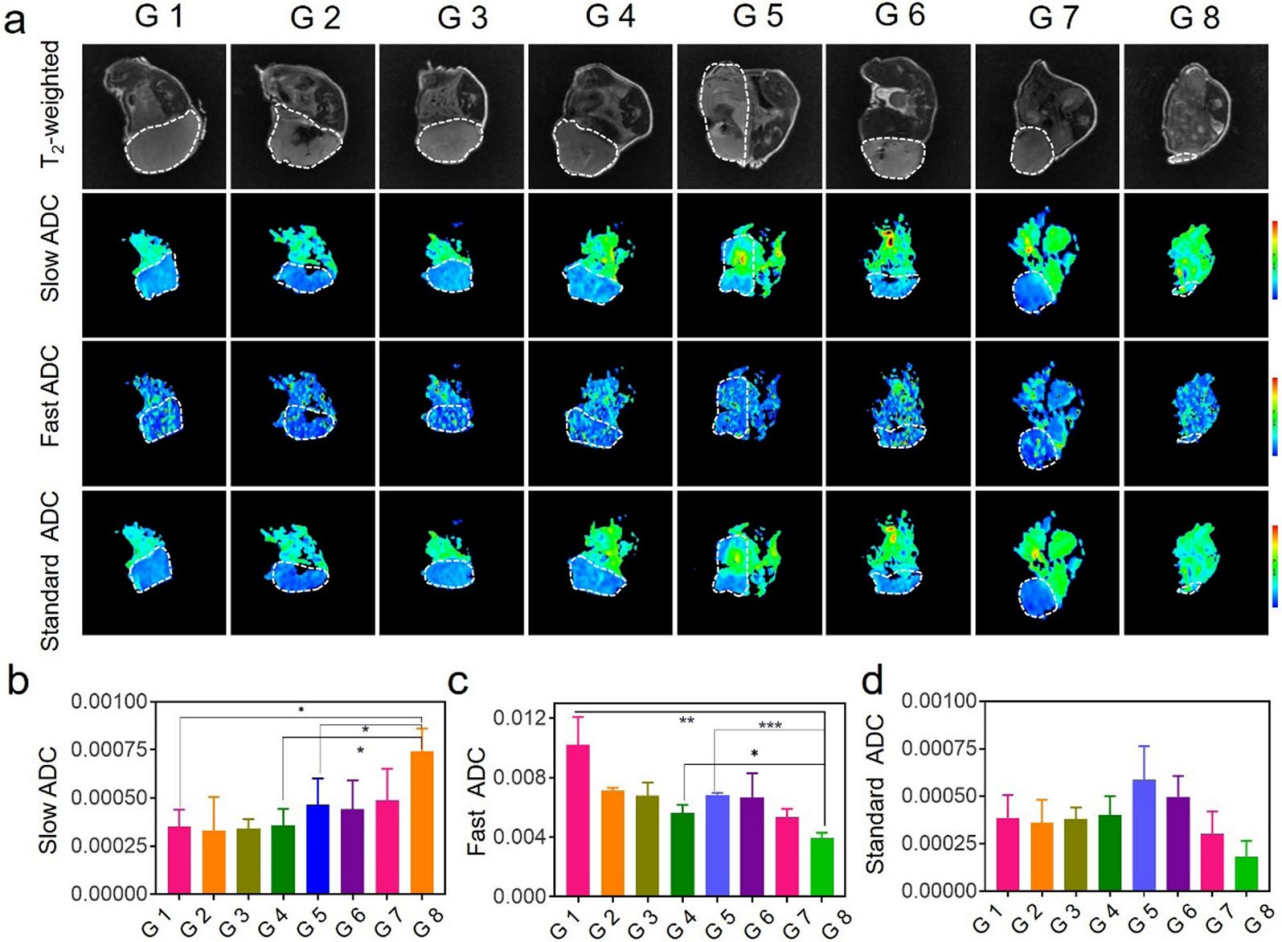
T_2_-weighted imaging analysis of tumor regions of different treatments after 21 days. a Images of T_2_-weighted MRI, fast ADC, slow ADC and standard ADC of EJ bladder tumor model with different treatments at 21 days. b Values of slow ADC. c Value of fast ADC. d Value of standard ADC. G 1: saline, G 2: FeSe_2_-PEG; G 3: BPs-PEG; G 4: BPs-FeSe_2_-PEG; G 5: laser; G 6: FeSe_2_-PEG + Laser; G 7: BPs-PEG + Laser; G 8: BPs-FeSe_2_-PEG + Laser. Data are presented as means ± SD (n = 3), (P* < *0.001, P* < *0.01, or *P* < *0.05)*
